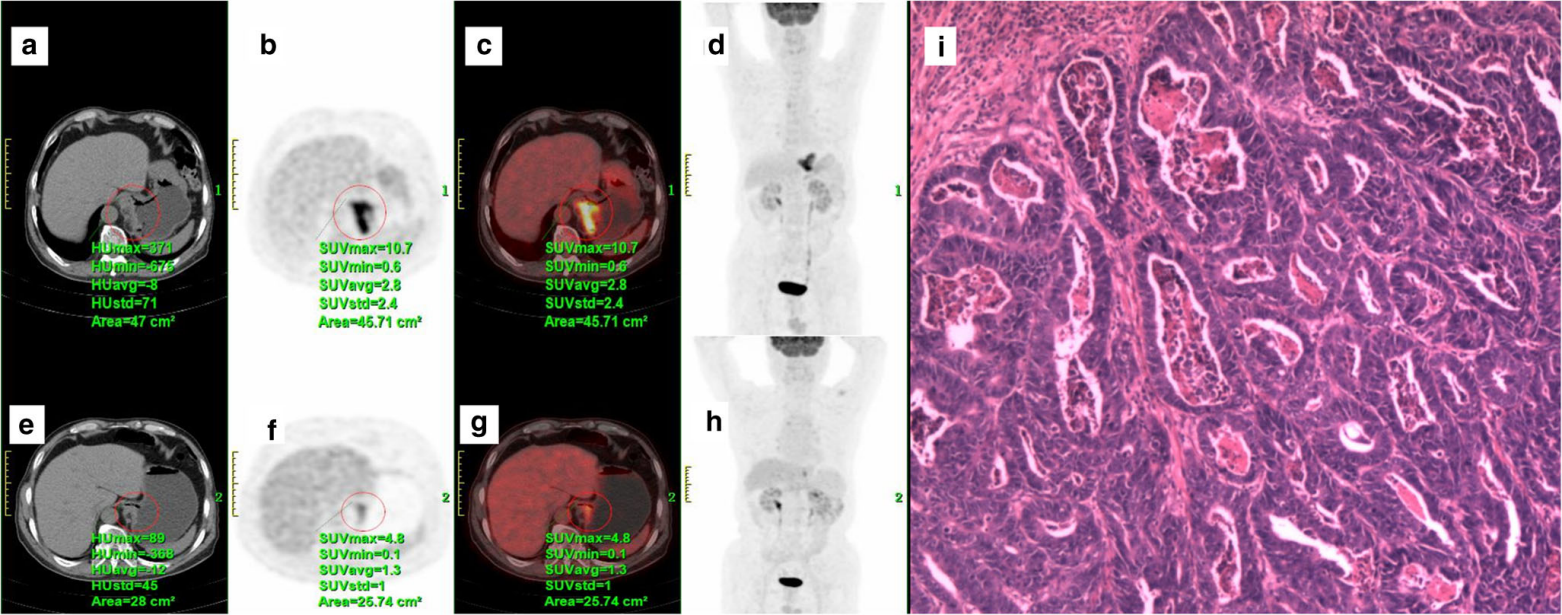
^18^F-FDG PET/CT scan of a 72-year-old man with gastro-oesophageal junction cancer (a–d) demonstrated by an intense uptake of ^18^F-FDG before treatment (SUV_max_ = 10.7) (c). After two cycles of chemotherapy (paclitaxel + cisplatin + fluorouracil) (e–h), the SUV_max_ of the lesion decreased to 4.8 (g), showing good response to the therapy. Final pathology (i) ypT3N0 (tumour regression grade 1)
